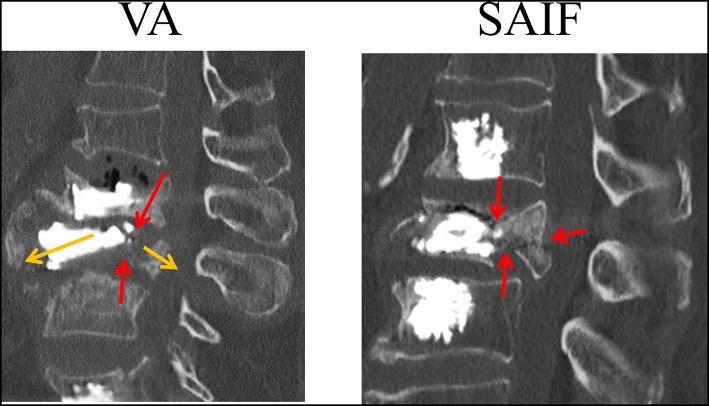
CT image (sagittal slices) taken on a lumbar vertebrae that re-fractured following VA (courtesy of A.C.). The L3 vertebra, previously treated with VA re-fractured with splitting of the anterior and middle column (yellow arrows); it can be noticed that a continuous fracture spreads from the superior to the inferior endplates (red arrows) with posterior wall retropulsion.
